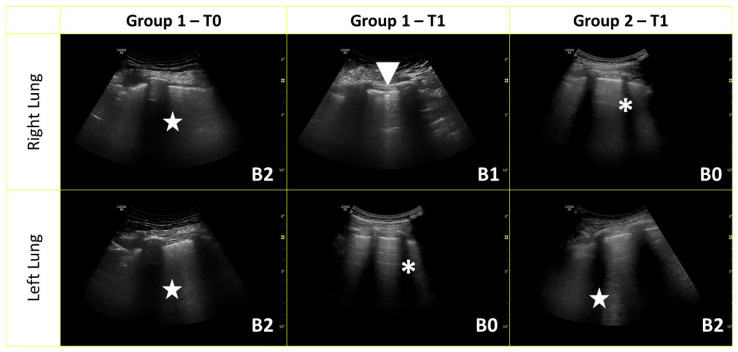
Representative images from one patient for each group (at the different time points) from the right and left lungs used to evaluate the lung ultrasound (LUS) score. The score for each segment is reported at the bottom of each panel. Multiple B-line conglomerations are indicated with (★), B-lines with little subpleural consolidation are indicated with (▼) while (✱) indicates A-lines.
